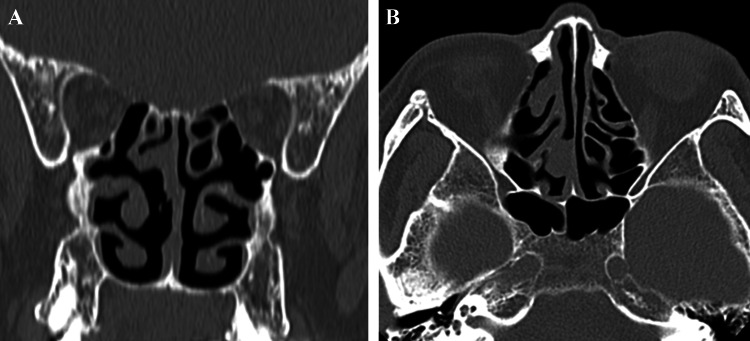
a and b A computed tomography scan showed a small mass in the right nasal cavity that developed from septal wall
